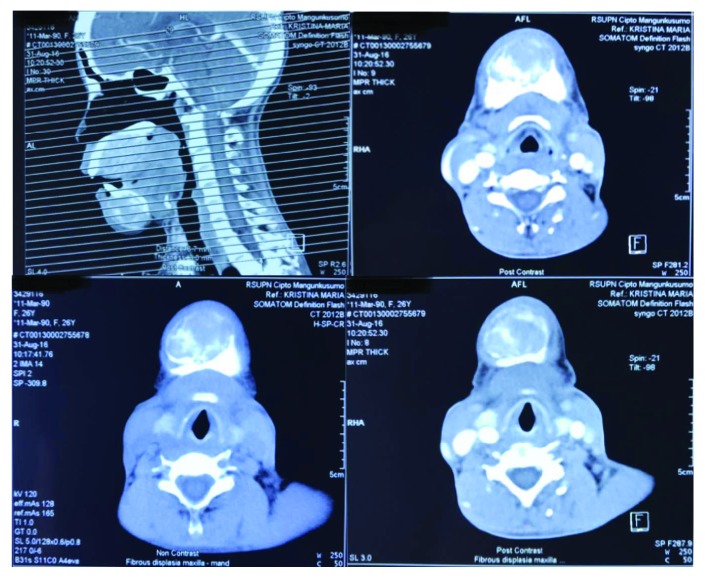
Case 1. Paranasal CT scan. Decreased diffuse with ground glass appearance among the cranium, facial, and cervicothoracic bones. Multiple lytic lesion in the maxillary bones.
